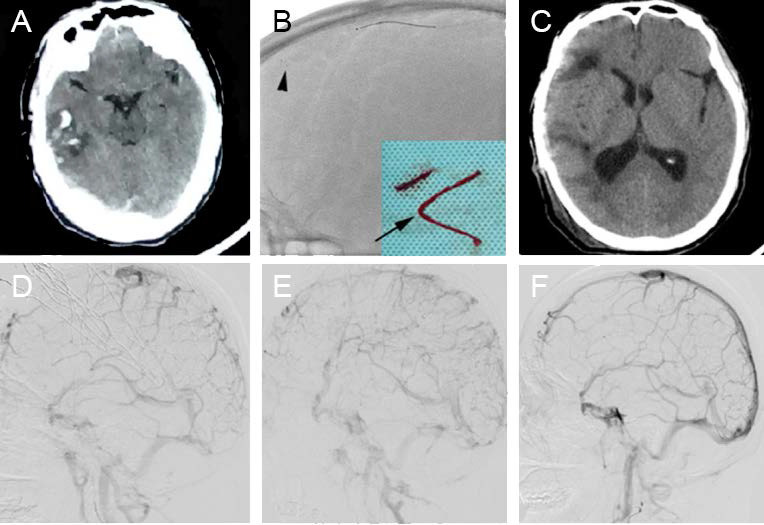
(A) A 47-year-old male patient presented with a headache and worsened in the next day. The computed tomography (CT) scan revealed right temporal lobe hemorrhage. (B) Stent retriever thrombectomy was performed. The arrow indicates the Solitaire FR stent deployed in the occluded superior sagittal sinus (SSS). The arrowhead indicates the clots that were removed. (C) The 4-month follow-up CT scan was normal, apart from the cranioplasty. (D) The lateral digital subtraction angiography (DSA) sinus phase image of the right side demonstrated extensive severe cerebral venous sinus thrombosis. (E) DSA revealed partial recanalization of the occluded sinus. (F) DSA revealed complete recanalization of the SSS with normal arteriovenous blood flow in the left transverse sinus at the 4-month follow-up. Reproduced with permission from Spandidos publisher (journal citation^76^). Copyright: © Wang et al.
